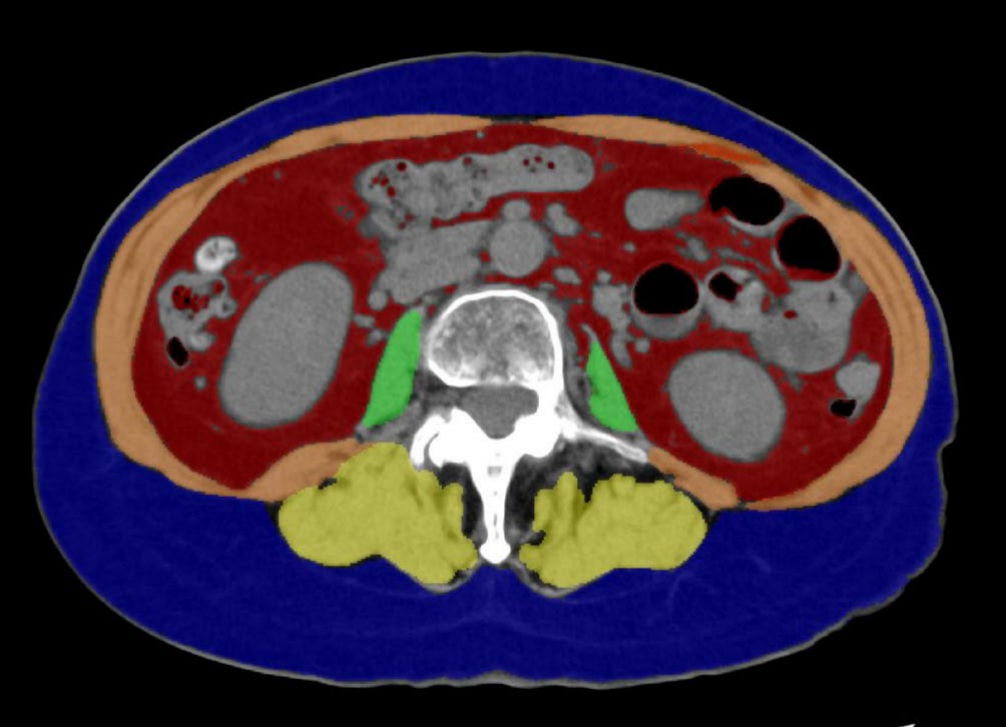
Body composition indices in computed tomography images taken at the level of the third lumbar vertebra. The green, orange, and yellow areas represent skeletal muscles. Specifically, the green area indicates the psoas muscle, the red area represents visceral adipose tissue, and the blue area represents subcutaneous adipose tissue.
